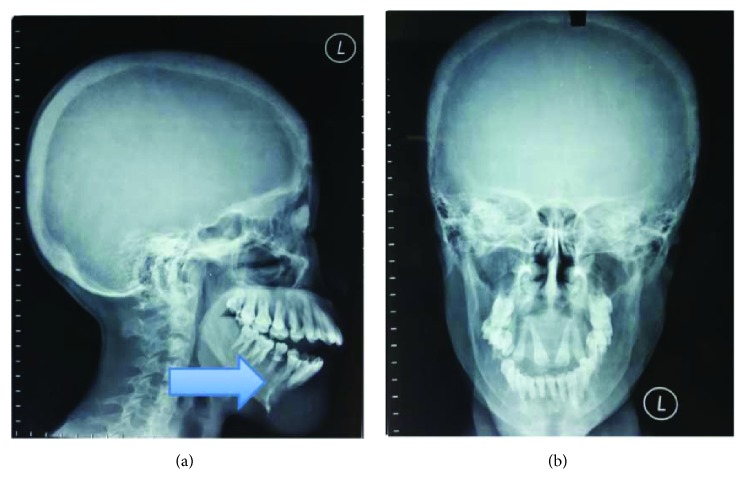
Case 1. (a) Bone survey radiographic examination of the cranium. (b) Multiple lytic lesion with well-defined border in the cranium. Arrow shows bone resorption activity in lower alveolar area.
